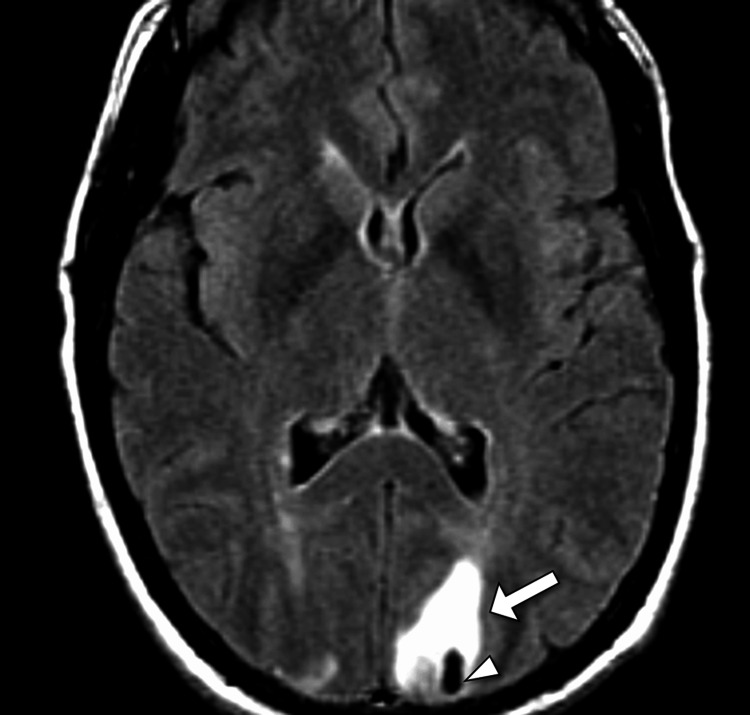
Axial MRI FLAIR showing parietal hyperintensity and hemorrhage in posterior reversible encephalopathy syndrome Axial magnetic resonance imaging (MRI) using fluid-attenuated inversion recovery (FLAIR) demonstrates a hyperintense signal in the left parietal lobe (arrow). A corresponding blooming artifact on susceptibility-weighted imaging (arrowhead) indicates hemorrhage. These features are characteristic of posterior reversible encephalopathy syndrome (PRES) with hemorrhagic involvement.
